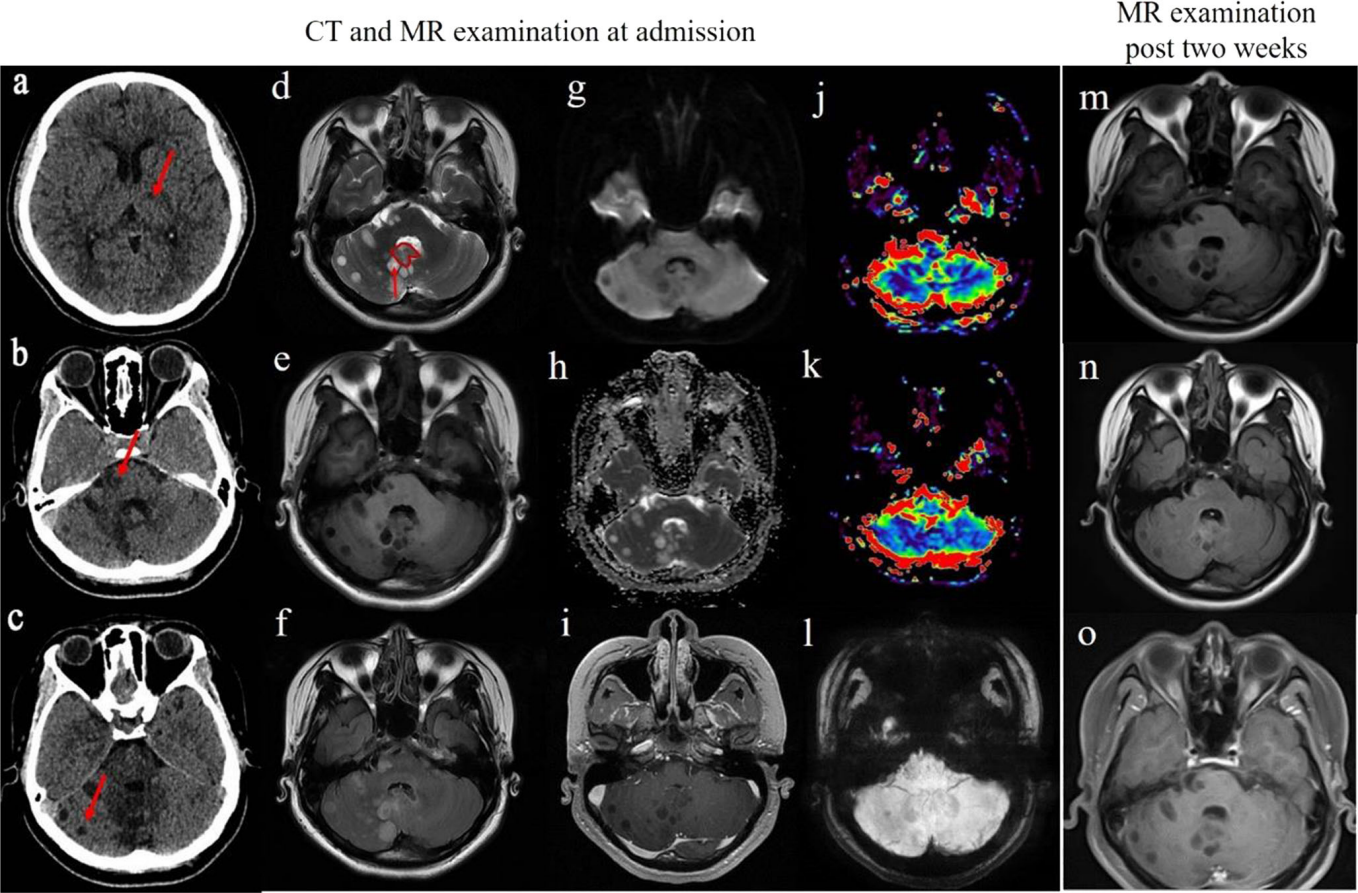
a–c CT findings show the tumors in left thalamus, brain stem, and bilateral cerebellar hemisphere (red arrow). d–l MRI findings. The lesions in bilateral cerebellar hemisphere. d Hyper-intense in T2-weighted image and small nodule-like higher signal (red arrow) along with circled solid component. e Hypo-intense in T1-weighted image. f T2 flair image showed iso-hyper intense. g Hypo-intense in DWI. h Hyper-intense in ADC. i No obvious enhancement majority and minority presented as mild annular enhancement. j–k Perfusion-weighted imaging color map, decreased regional cerebral blood flow, and regional cerebral blood volume. l minor hemorrhage in the lesions. l SWI showed minor hemorrhage. m–o MRI findings for post 2 weeks. m–o Corresponding T1WI, T2 flair, and T1WI enhancement, no obvious changes compared with before
